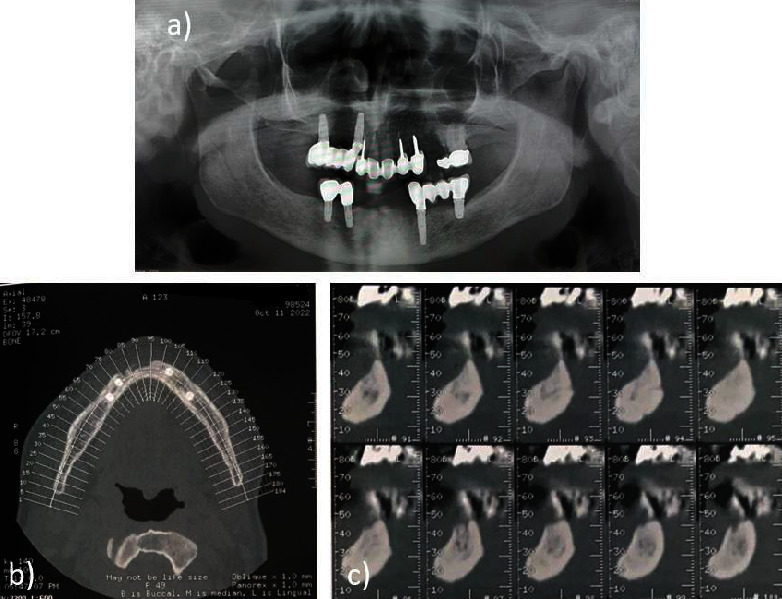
Radiographic examinations performed in October 2022: (a) the OPT showed an absence of radiolucency in the region of the lower incisors; the CBCT showed a complete healing of the bone tissue; (b) axial cuts of CBCT; (c) sagittal cuts of CBCT.
